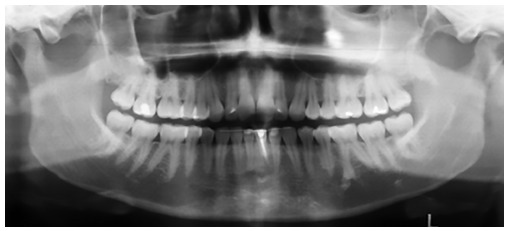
Long-term post-treatment (T_3_) panoramic radiograph.
